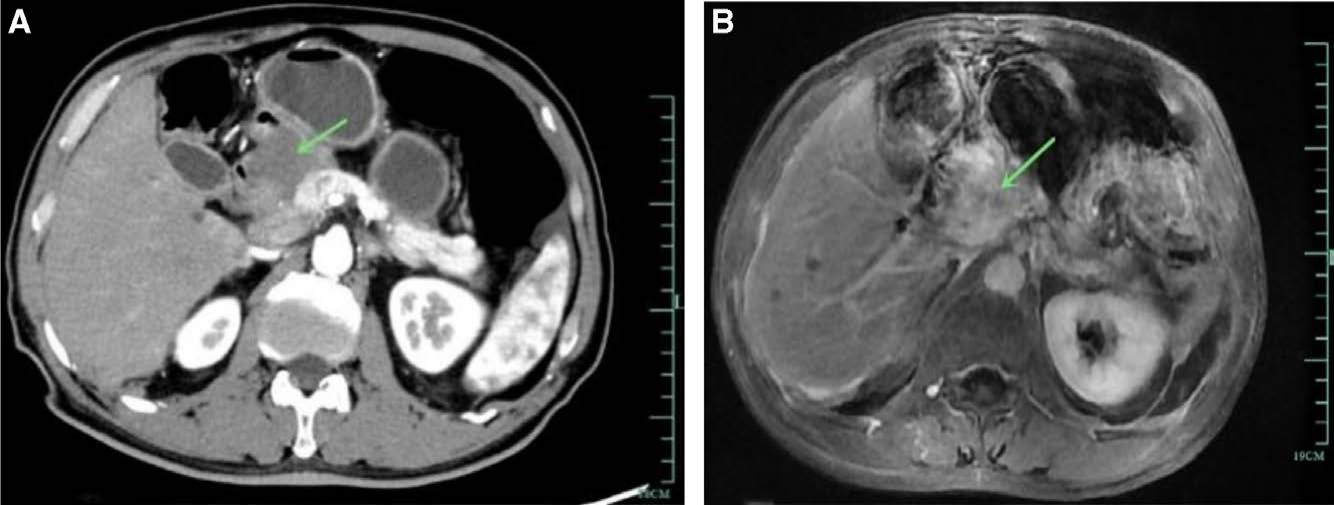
(A) The enhanced CT scan reveals a tumor in the head of the pancreas, with possible invasion of the pylorus and duodenum, and gastric retention with dilation. (B) The enhanced MRI shows tumor invasion of the bile duct. CT = computed tomography, MRI = magnetic resonance imaging.
